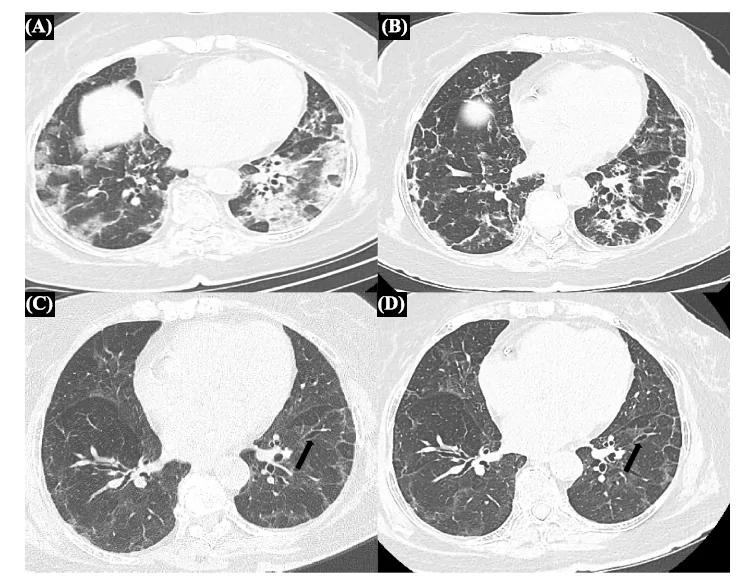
Imaging findings in an 82-year-old female with persistent pulmonary fibrosis. (A) Unenhanced axial CT during acute infection (10 days after symptom onset) reveals bilateral diffuse ground-glass opacities and partial consolidations. (B) Follow-up unenhanced axial CT at 28 days post-infection shows partial resolution of bilateral pulmonary lesions. (C, D) CT images obtained at 8 and 12 months post-infection display localized bronchiectasis (black arrows) in the anteromedial basal segment of the left lower lobe, with residual ground-glass opacities and reticular patterns in the background.
